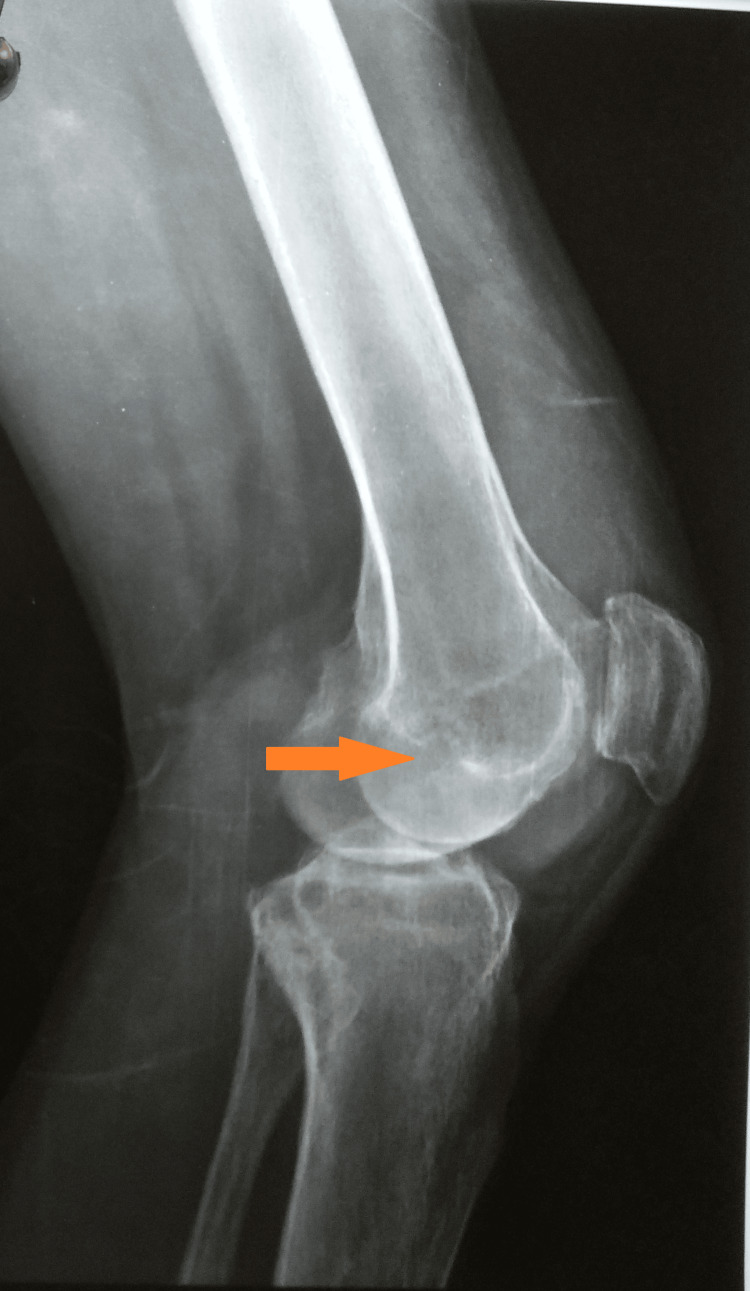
Plain radiograph of the left knee (lateral view) suggestive of tibial involvement
